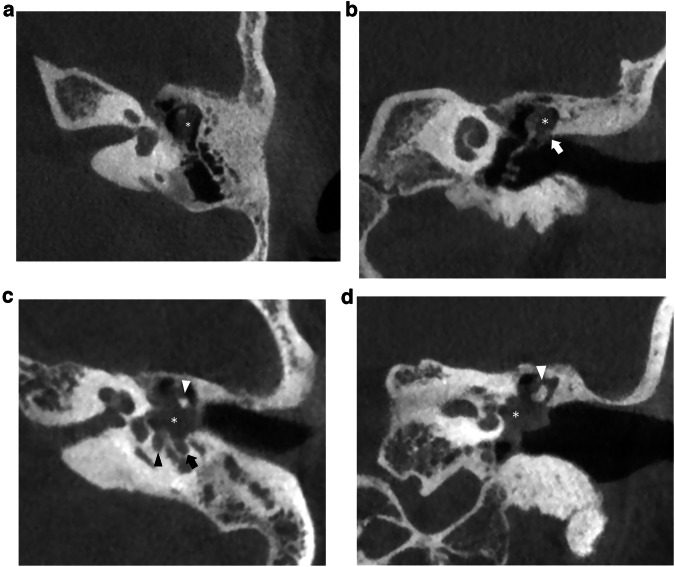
Axial (a) and coronal (b) CBCT images of a typical pars flaccida cholesteatoma in the left temporal bone extending in Prussak’s space with medial displacement and erosion of the ossicular chain (asterisk in a, b). Notice the erosion of the scutum (white arrow in b) and the presence of a tympanostomy tube. Axial (c) and coronal (d) CT of a typical pars tensa cholesteatoma (asterisk in c, d), originating in the lower and posterior part of the middle ear, extending in the tympanic sinus (black arrowhead) and facial recess (black arrow). There is lateral displacement of the ossicular chain and erosion of the incudostapedial joint (white arrowheads in c, d) which should be visible on this coronal slice. Both these lesions and CC are often undiscernible on MRI
